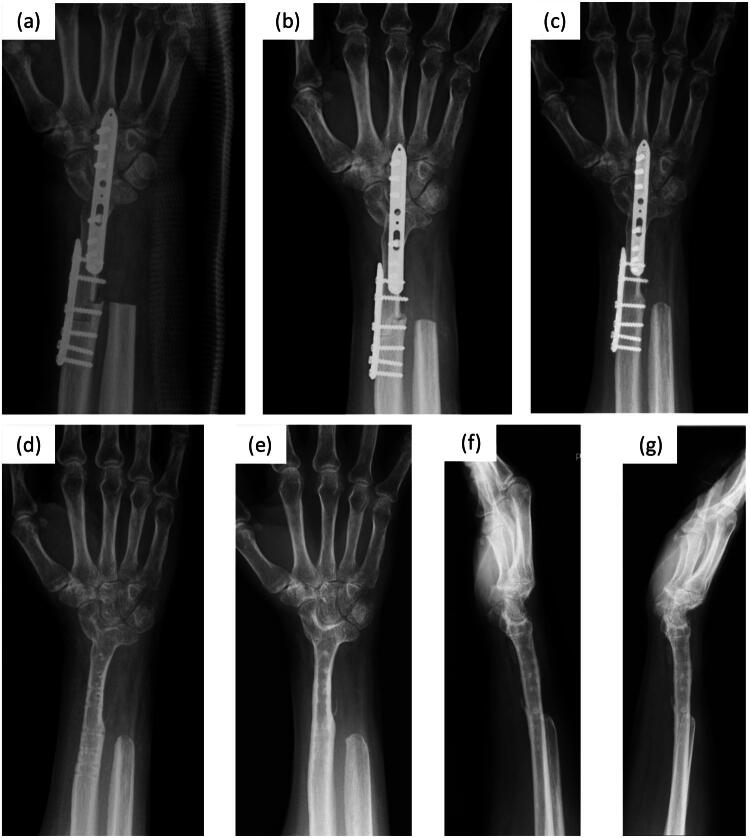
Postoperative radiographs. (a) After surgery. (b) Three months postoperatively. (c) Bone union is observed 9 months postoperatively. (d) After implant removal (9 months postoperatively). (e–g) Anteroposterior view (e), palmar flexion (f), and dorsiflexion (g) at 7 years postoperatively. Subtle wrist motions at the distal carpal alignment are observed.
